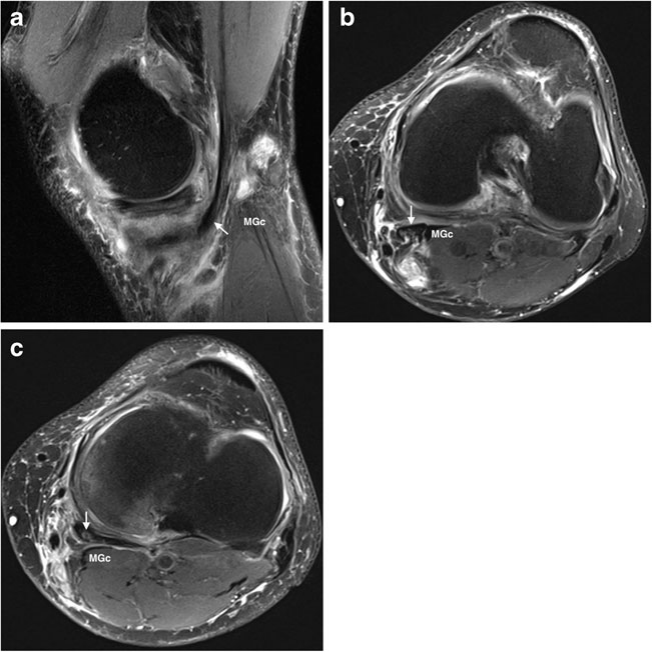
a-c. Ruptured Baker’s cyst in a 62-year-old man presenting with acute pain in the popliteal fossa and the medial side of the left leg after a run. Sagittal FS PD-weighted MRI (a) shows diffuse hypodermic edema and a popliteal cyst with irregular and undefined margins in close relation to the medial gastrocnemius muscle and the semimembranosus tendon (arrow). Axial FS PD-WI MRI along the proximal-to-distal axis (b and c) better demonstrates the intrasubstance edema of the semimembranosus tendon (arrows) so as to show the fluid tracking along the medial side of the leg, adjacent to the medial gastrocnemius muscle and the pes anserinus. MGc, medial gastrocnemius
